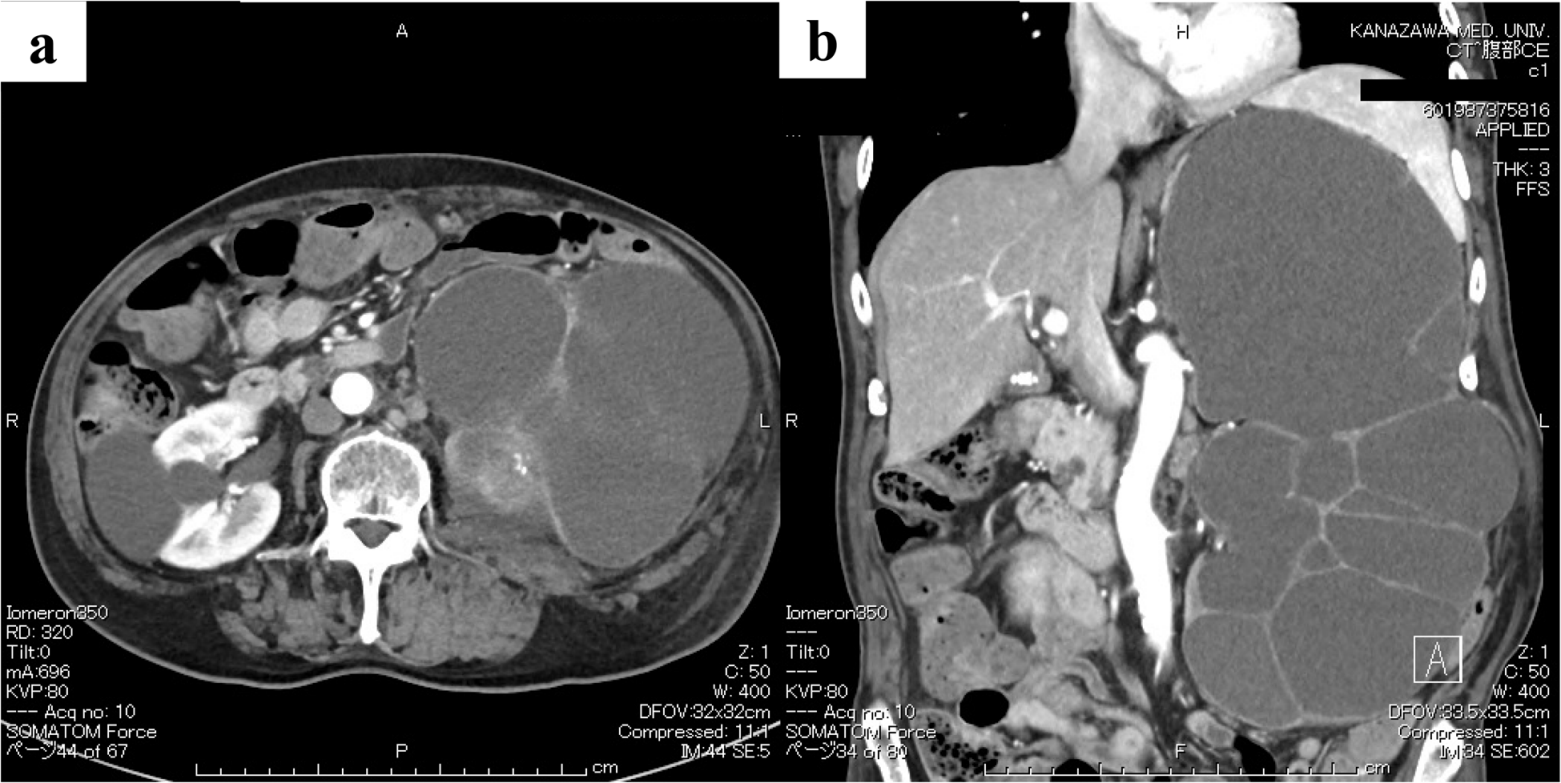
Computed tomography of the abdomen before nephrostomy. The left kidney was multicystic and markedly enlarged
